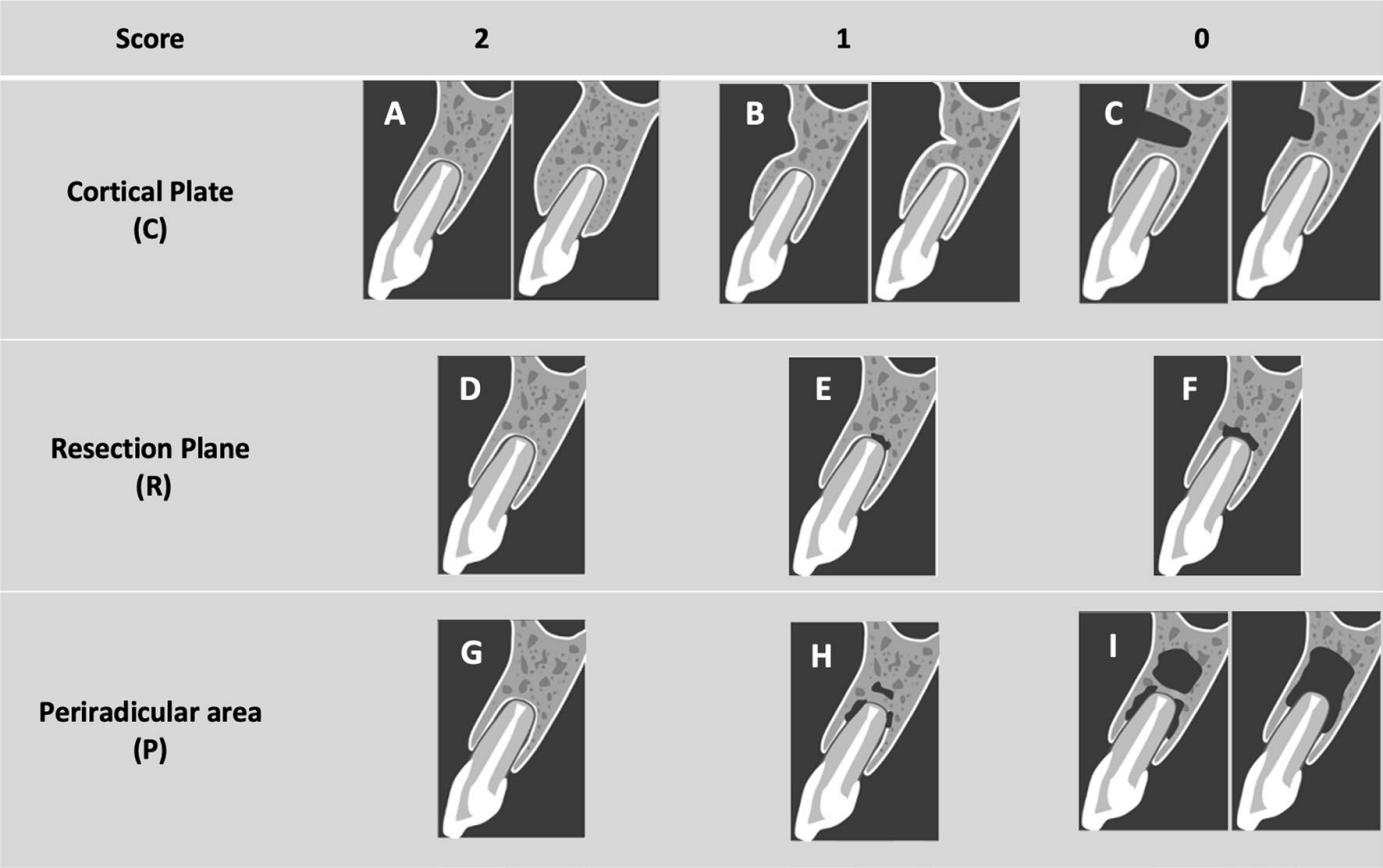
The modified Chen et al. criteria for 3-dimensional radiographic evaluation after endodontic microsurgery. Cortical plate (access bony window) C-index. (A) Score 2: the cortical plate is re-established and flat. (B) Score 1: the cortical plate is re-established, but concave or with minor indentations. (C) Score 0: the cortical plate has not been re-established or is only partially re-established. Resection plane (cut root face) R-index. (D) Score 2: complete bone deposition on the resection plane (complete formation of periodontal ligament [PDL] space of normal width). (E) Score 1: partial bone deposition on the resection plane (partial formation of PDL space). (F) Score 0: no bone deposition on the resection plane (no formation of PDL). (G) Periradicular area P-index. (G) Score 2: complete bone formation in the periradicular area. (H) Score 1: partial bone formation in the periradicular area that appears to be smaller than the lesion size in the preoperative cone-beam computed tomography regardless of the R or C indices. (I) Score 0: no apparent bone formation around the periradicular area regardless of the R or C index
